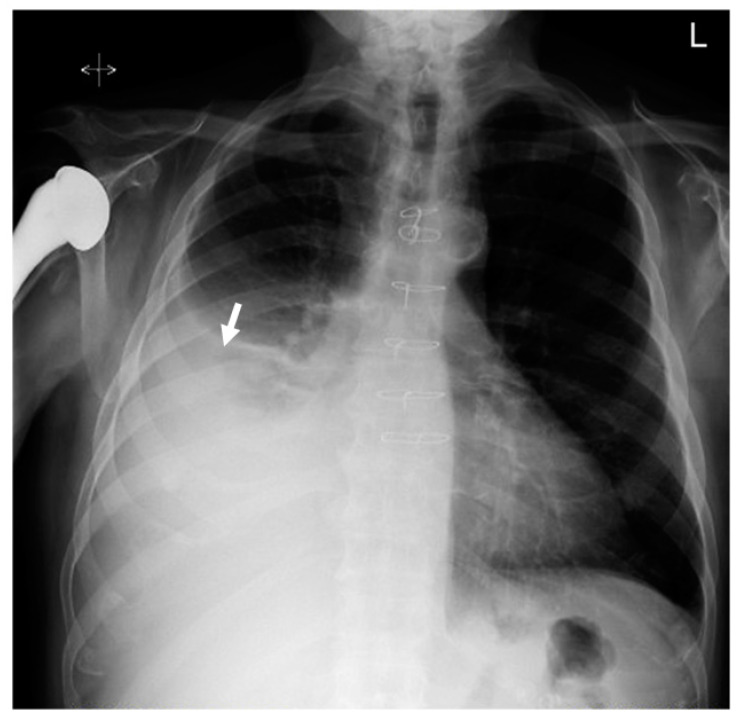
Chest X-ray demonstrating stable large right-sided pleural effusion (white arrow) with associated atelectasis/consolidation.
